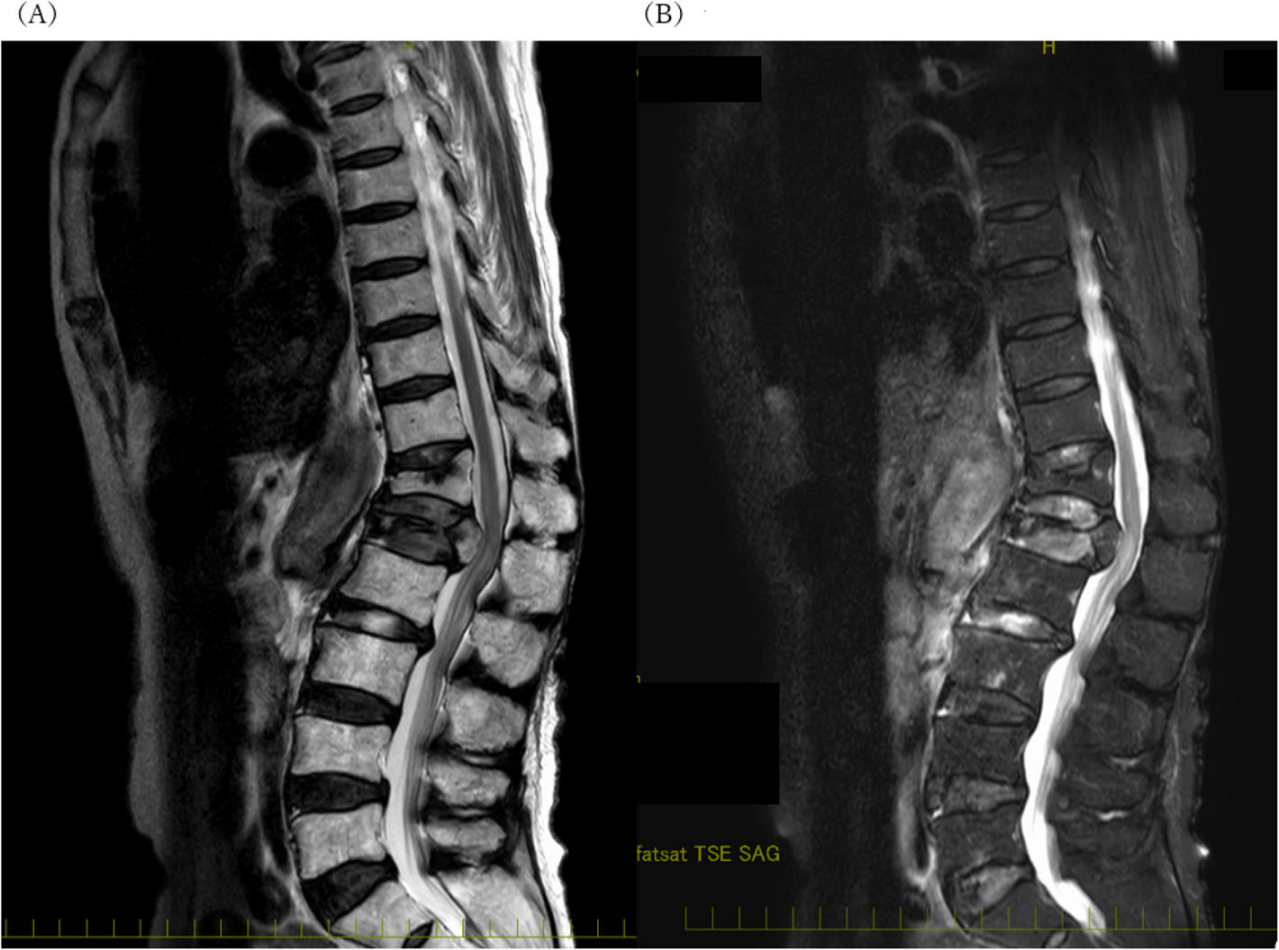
Magnetic resonance imaging on postoperative day 1. A A sagittal, T2-weighted image revealed the compressed spinal cord at the L1 level. B A sagittal, fat-suppression, T2-weighted image revealed a low intensity of the T12 vertebra and a high intensity of a portion of the L1 vertebra
